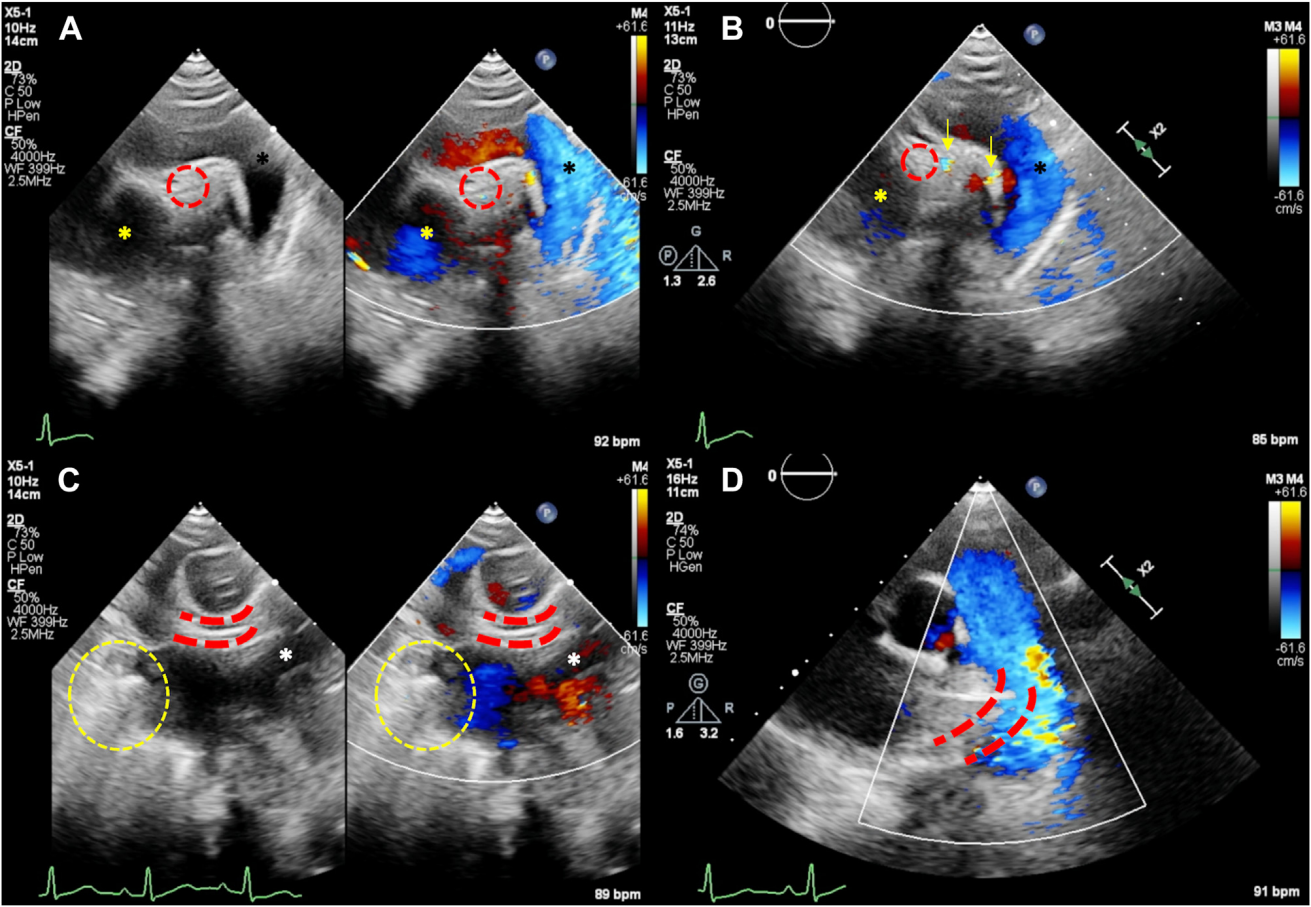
Two-dimensional TTE without (*left*) and with (*right*) color-flow Doppler, suprasternal view (A), demonstrates the LA (*yellow asterisks*), descending aorta (*black asterisks*), and absence of the RPA, which should be located where the *red dotted circles* are placed. (B) There is evidence of flow within the left main bronchus (*yellow arrows*) suggestive of collateral blood flow (later confirmed to be bronchial artery collateral flow on CT imaging). (C) From the crab view window, the left pulmonary veins (*white asterisks*) were visible, but there was absence of the right pulmonary veins, which should be located where the *yellow dashed circles* are placed. The RPA was also absent, which should be located where the *red dotted lines* are placed. (D) On the high parasternal short-axis view, there was missing divergent flow from the main pulmonary artery to the RPA, which should be located where the *red dotted lines* are placed.
